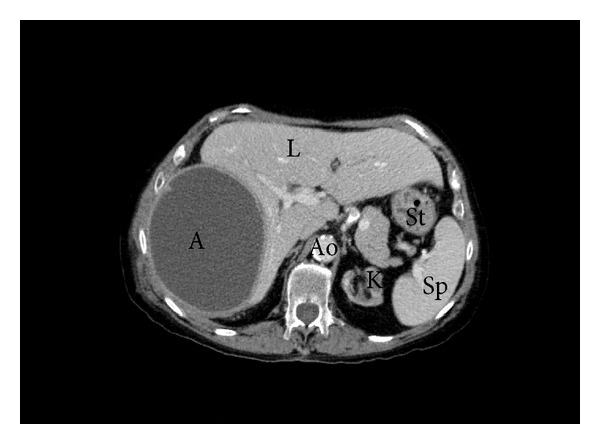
Computed tomography showing a 13 × 10 × 16 cm abscess (A), compressing the liver (L); abdominal aorta (Ao); kidney (K); spleen (Sp); stomach (St).
